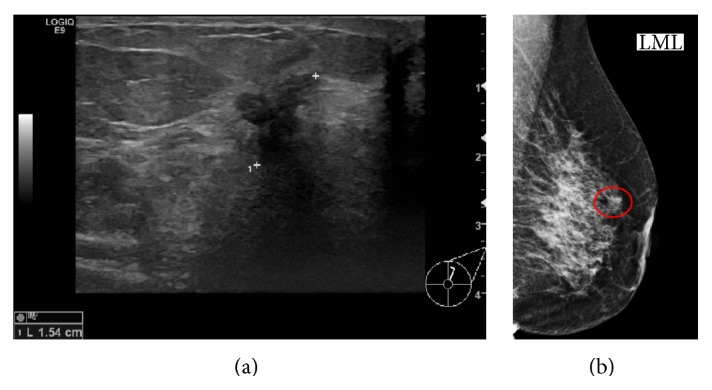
(a) Ultrasound of the left breast showing a 15 mm ill-defined hypoechoic mass. (b) Mammogram of left breast (mediolateral view) demonstrates a spiculate mass (red circle).
